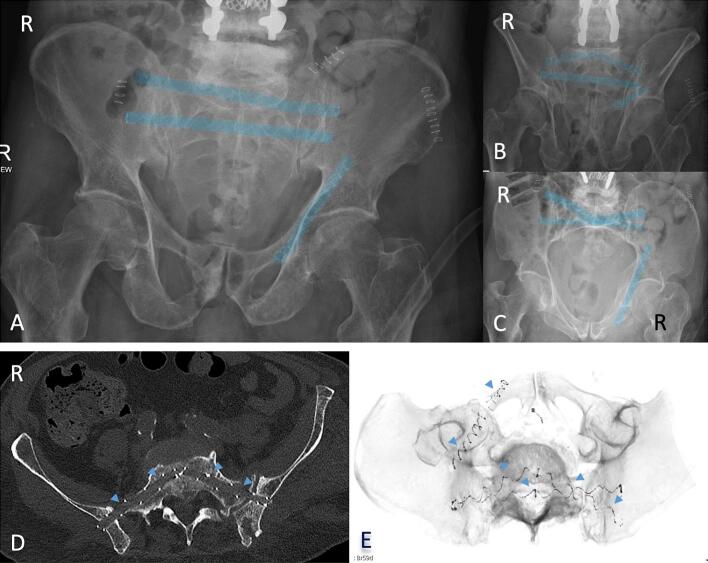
postoperative X-rays of the patient showing the radiopaque spiral of the implant. A: a.p. view of the pelvis, B: outlet-view of the pelvis, C: inlet-view of the pelvis; D: postoperative axial CT-scan, the photodynamic nail is marked by blue arrows; E: 3D-reconstruction of the postoperative CT-scan, all photodynamic implants are marked by blue arrows. (For interpretation of the references to colour in this figure legend, the reader is referred to the web version of this article.)
